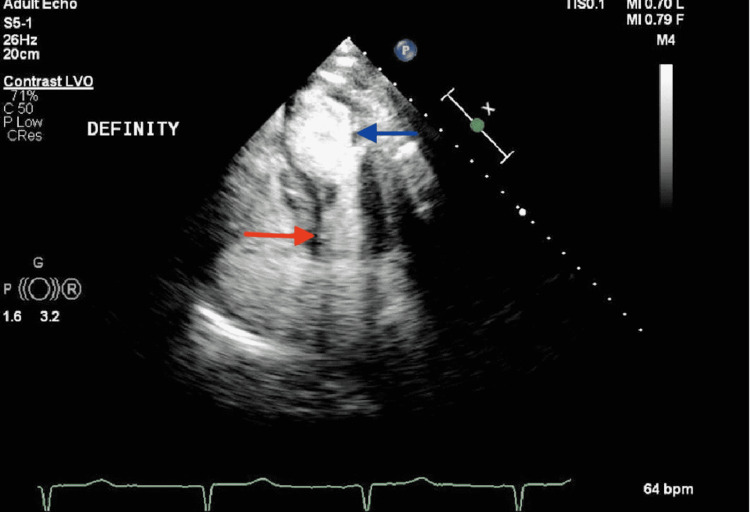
2D Echo on a patient with Takotsubo cardiomyopathy. 2D echocardiography (Echo) with contrast, imaged during systole depicting normal contraction at the base depicted by the red arrow and severe hypokinesis at the apex depicted by the blue arrow.
